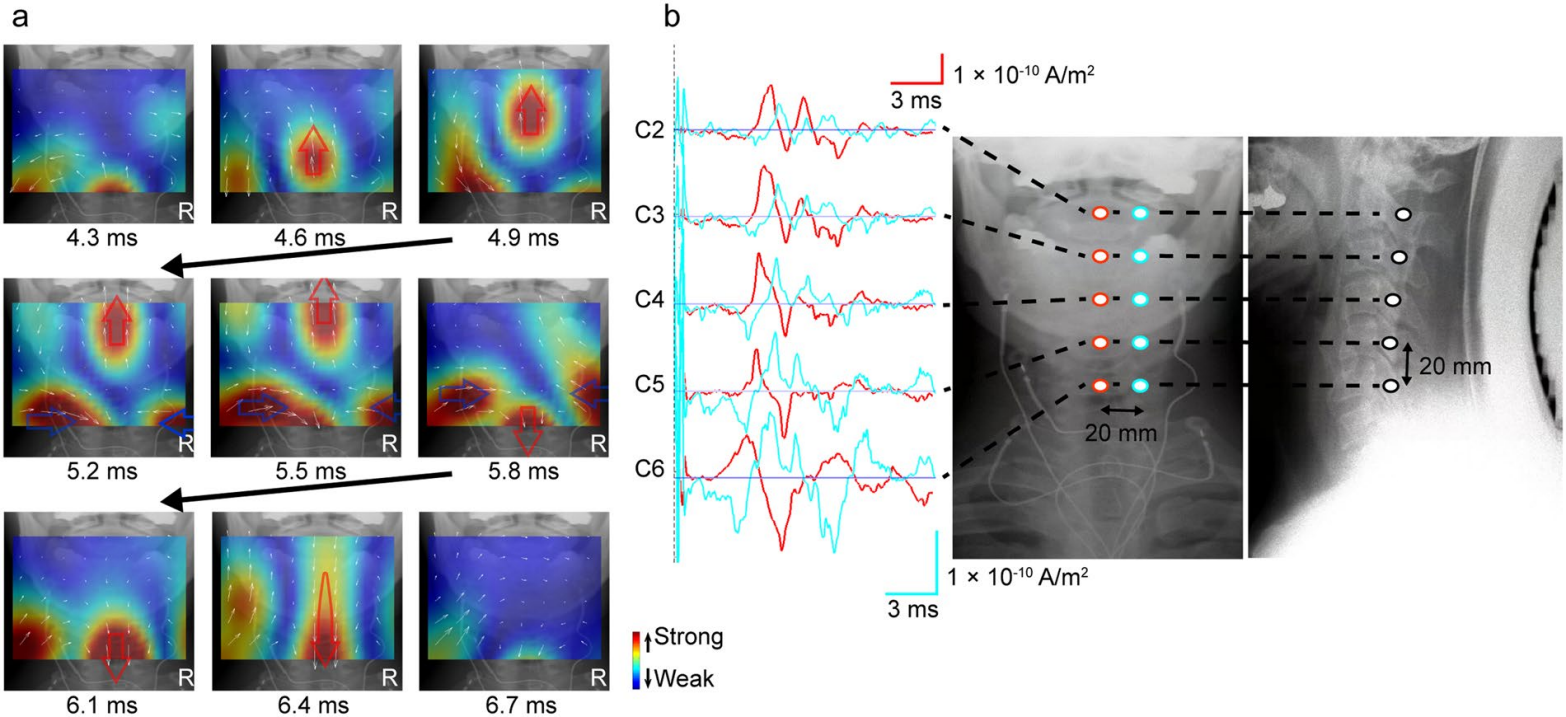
Reconstructed currents of a healthy subject measured after stimulation of the lower thoracic spinal cord. (a) Reconstructed current map. Currents at the level of the spinal canal were reconstructed by a recursive null-steering (RENS) beamformer and superimposed on an X-ray image. Current intensity is shown by a colour scale (red is higher). Small white arrows indicate current vectors. The leading component of the currents (large upwards-pointing red arrow) appeared 4.3 ms after stimulation and propagated cranially along the spinal canal. At 5.8 ms, the trailing component (downwards-pointing red arrow) appeared from the caudal side. Perpendicular currents (blue arrows) flowing towards the spine between the leading and the trailing components are also observed on both sides. (b) Waveforms of reconstructed currents at the “virtual electrodes”. Red traces are currents at the midline of the cervical spinal canal (the caudal to cranial direction is upwards). Blue traces are currents 20 mm lateral from the midline (upwards is towards the spinal canal).
